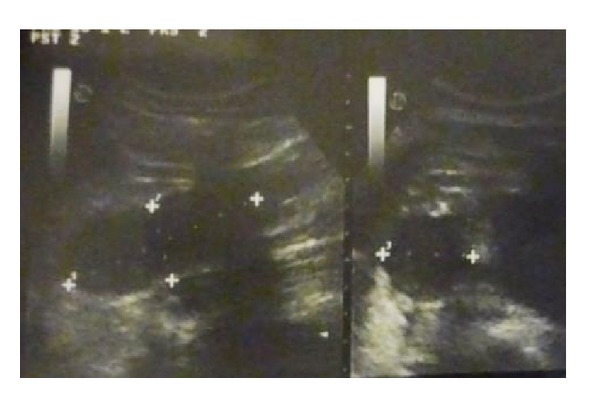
Sonographic images revealed a well-defined mass with lobulation and hypoechoic content in the posteroinferior of the right kidney.
